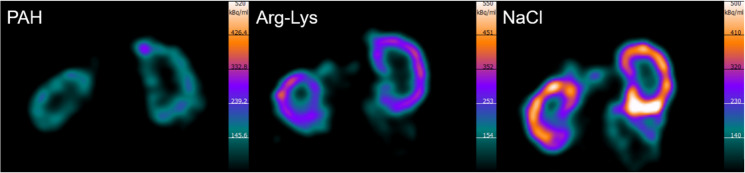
Exemplary SPECT images of kidney coronal sections 8 h after injection of [^177^Lu]Lu-DOTATOC in combination with paraaminohippurate (PAH), Arg-Lys, or 0.9% NaCl solution. Images indicate reduced radioactivity accumulation in renal cortex. Lighter color shows higher level of activity.
